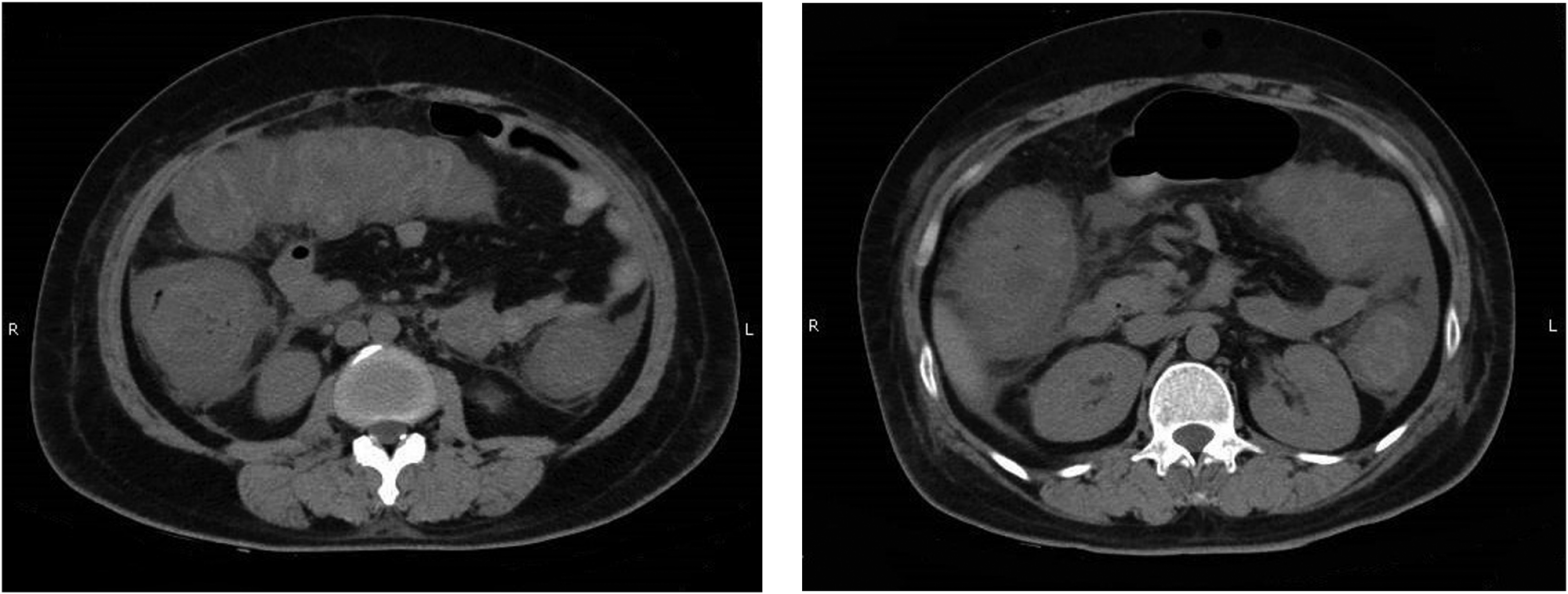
CT scan findings on August 7, 2022. These scan images showed thickening and swelling of the colorectal wall, abdominal and pelvic effusion, and a blurred abdominal fat space.
